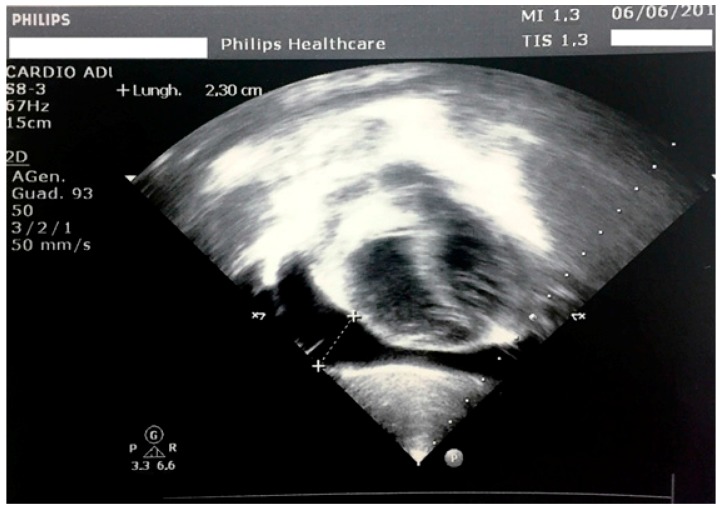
Echocardiography at admission, with evidence of a hyperechogenic pericardium and a large pericardial effusion.
